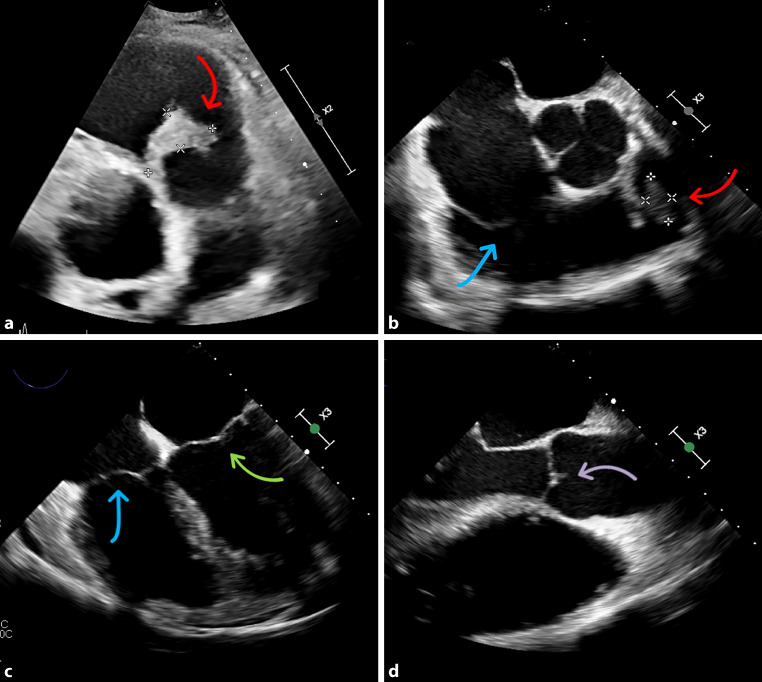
Vegetation at the pulmonary valve in transthoracic and transesophageal echocardiography. a Parasternal short axis view showing the vegetation on the pulmonary valve (*red arrow*). b Transesophageal echocardiography showing the vegetation at the pulmonary valve (*red arrow*) and the tricuspid valve (*blue arrow*) without any vegetation. c Transesophageal echocardiography showing the mitral valve (*green arrow*) and tricuspid valve (*blue arrow*) without any vegetation. d Transesophageal echocardiography showing the aortic valve without any vegetation (*purple arrow*)
